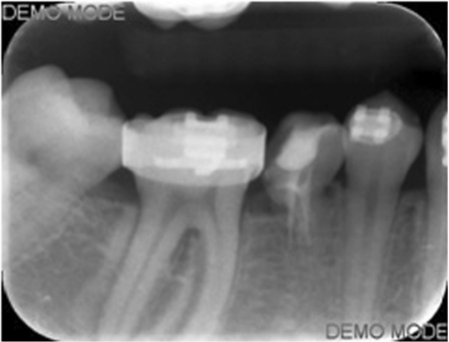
Radiograph 6 months post replantation showing almost total root resorption and complete space closure between the 1st permanent molar and 2nd primary molar
